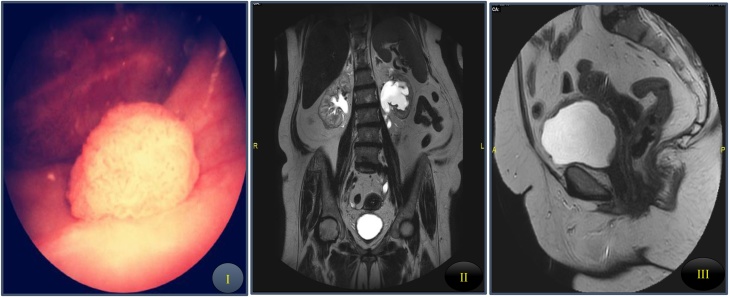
I. Cystoscopic examination revealed nodular mass at the trigone with smooth surface near left ureteric orifice. II and III. 3-Tesla MRI revealed average sized both kidneys with mild right hydronephrosis and left moderate hydronephrosis down to thickened bladder wall more at posterior bladder wall encroaching at both ureteric orifices. Bilateral external iliac lymphadenopathy and normal both ovaries.
